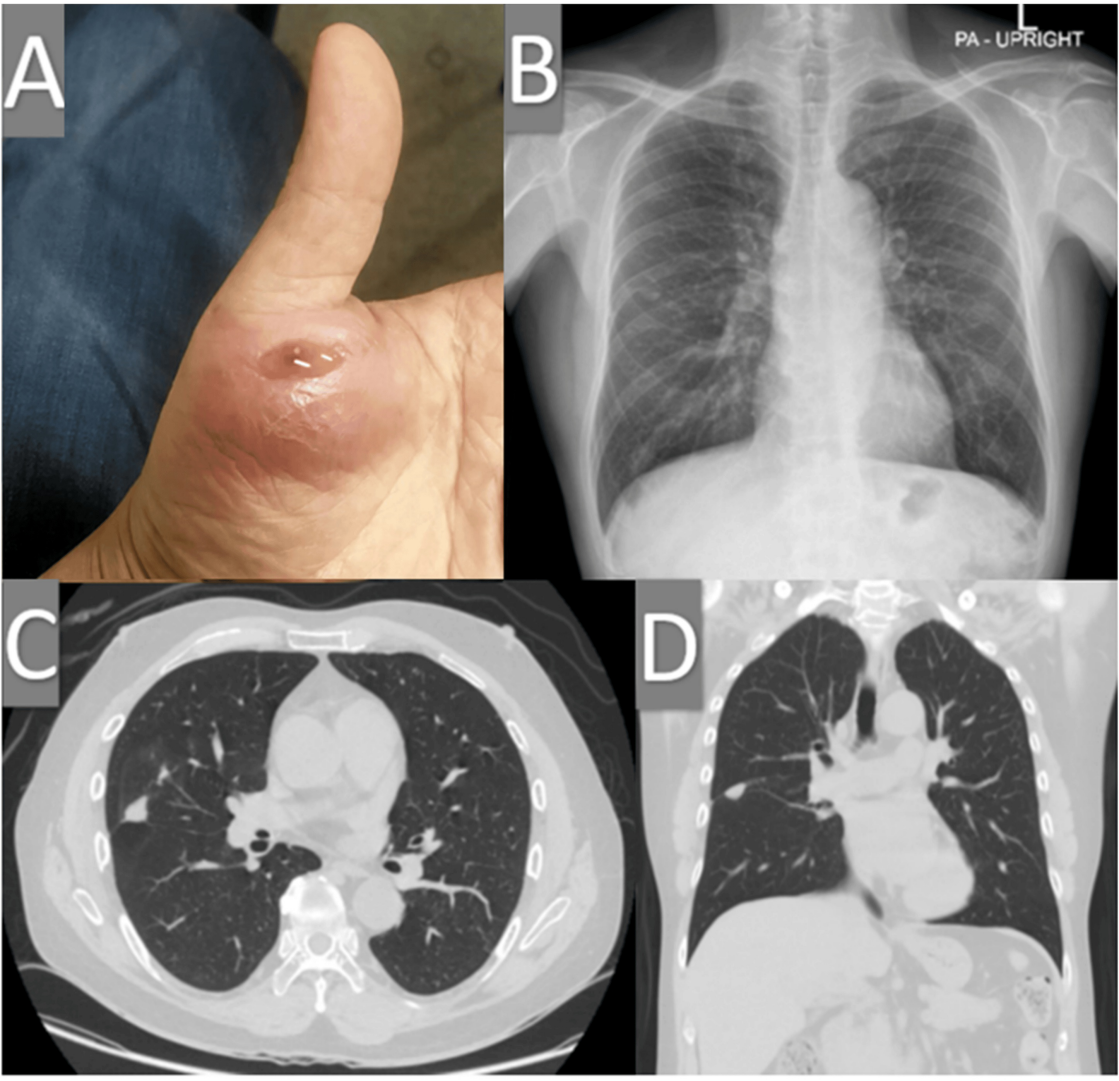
(A) Cutaneous abscess on the right thenar area with yellow pus accumulation. (B) Chest radiograph showing diffuse reticulonodular opacities with mass-like patchy infiltration in the right middle lung. (C, D) Chest CT images reveal a mass-like consolidation with surrounding centrilobular nodules and bronchiectasis in the lateral segment of the right middle lung.
